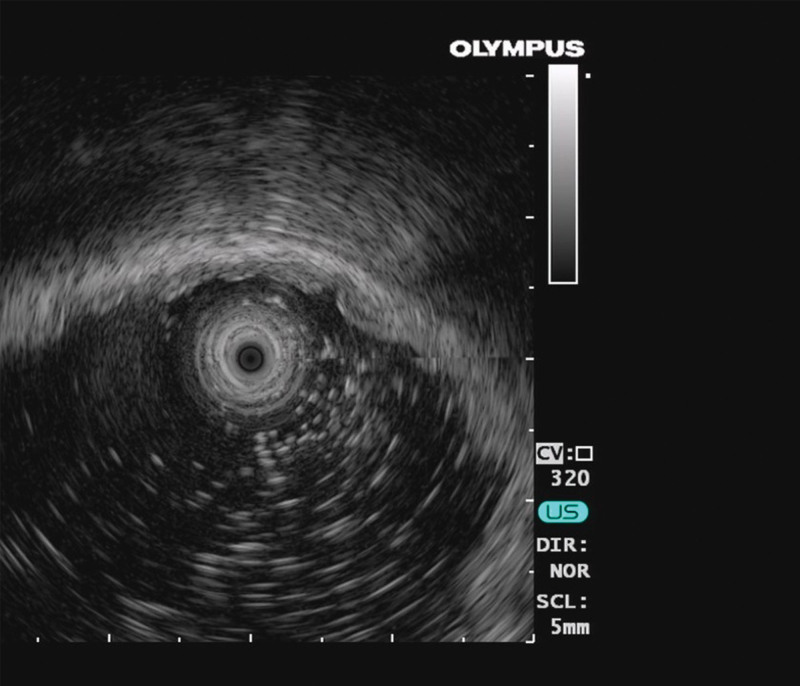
A clear structure of the gastric wall with a thickness of approximately 6.3 cm is observed using 12-MHz ultrasound. Both the mucosa and submucosa thickened, and the mucosa has a thickness of approximately 2.2 mm.
